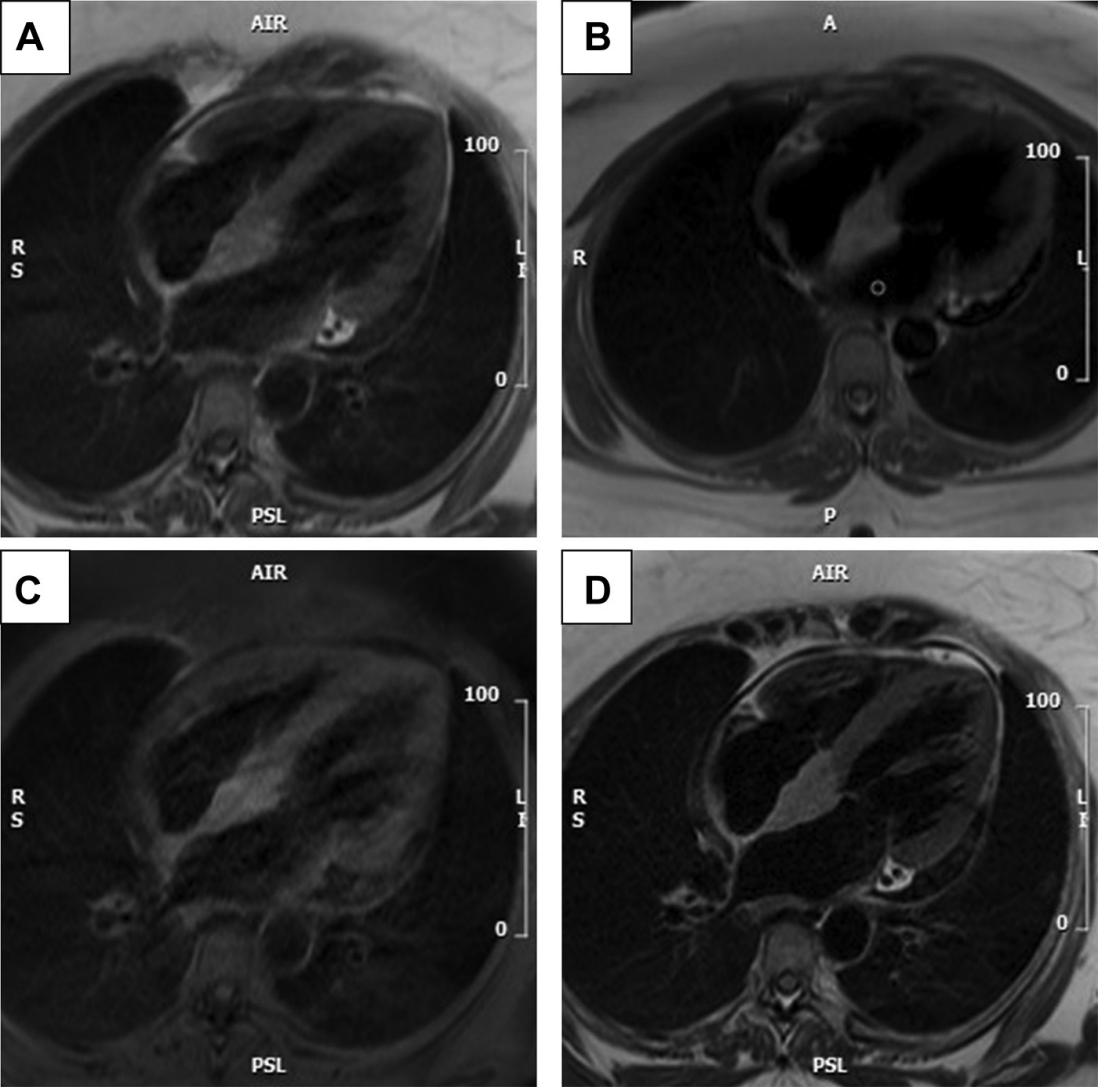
Noncontrast MRI Sequences (A) Half-Fourier Acquisition Single-shot Turbo spin Echo imaging showing a relative high signal in the IAS compared with myocardium; (B) T1 weighting showing hyperintensity in the IAS compared with myocardium; (C and D) T1 and T2 with fat suppression showing persistent high signal in the IAS. IAS = interatrial septum; MRI = magnetic resonance imaging.
